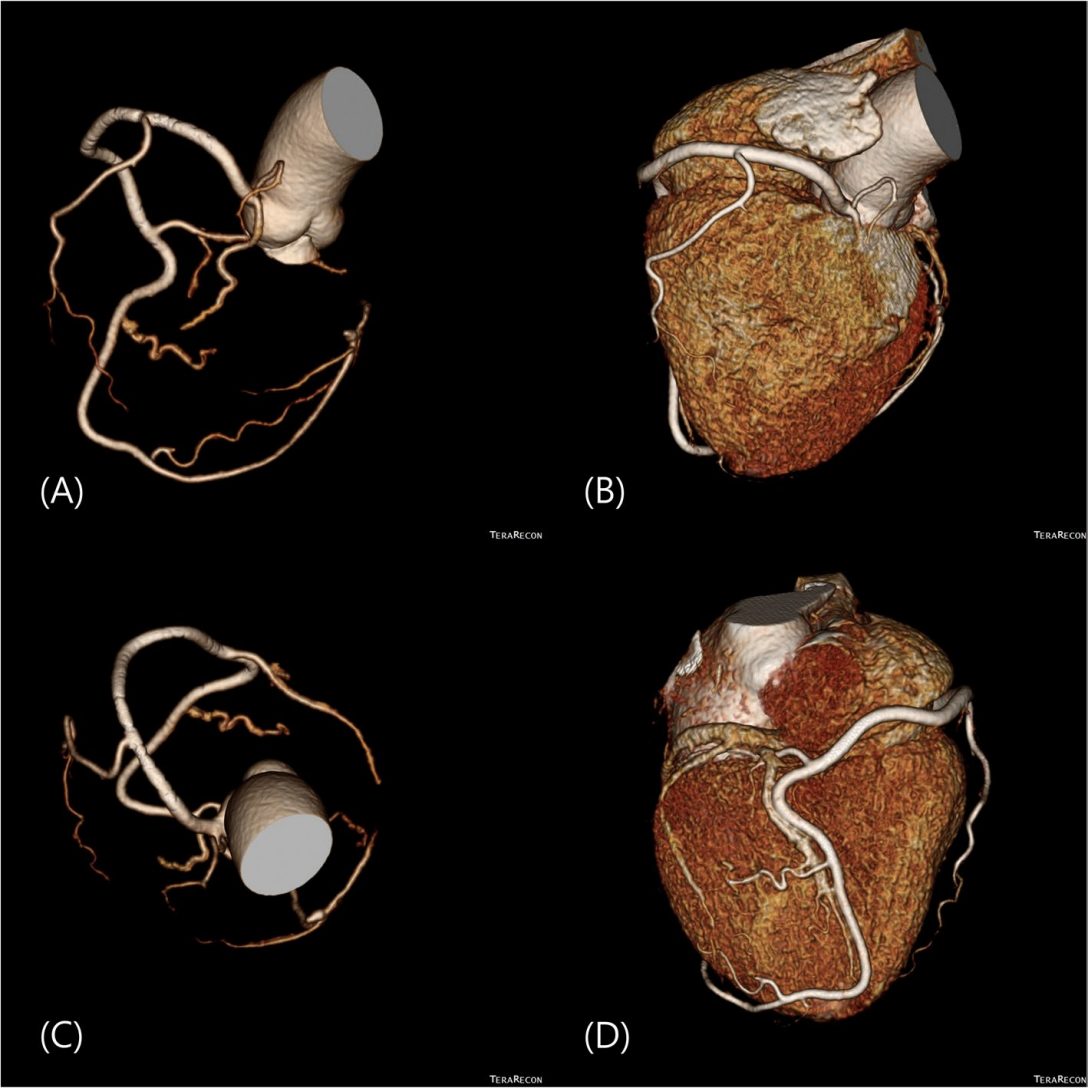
Coronary computed tomography angiography of the patient. A Volume-rendered coronary tree. B Volume-rendered reconstruction. C Cranial view of coronary tree (D) Posterior view of the coronary artery. The posterior descending artery courses through the heart’s apex and goes straight as the left anterior descending artery
